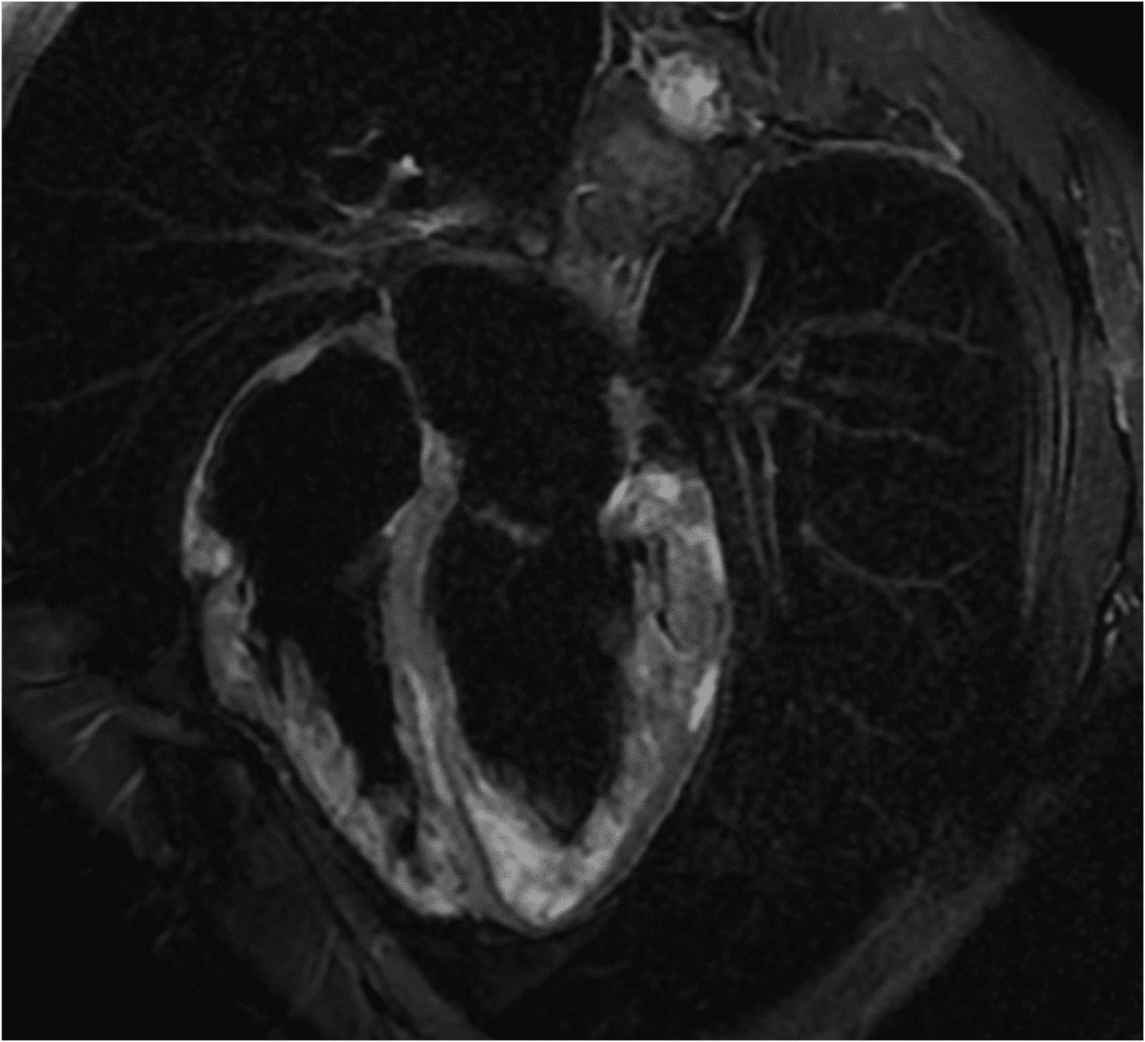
Cardiac MRI with gadolinium showing high T2 signal and late gadolinium enhancement in the pericardial region of basal inferolateral/inferior and all mid segments, consistent with non‐ischemic cardiac myopathy.
